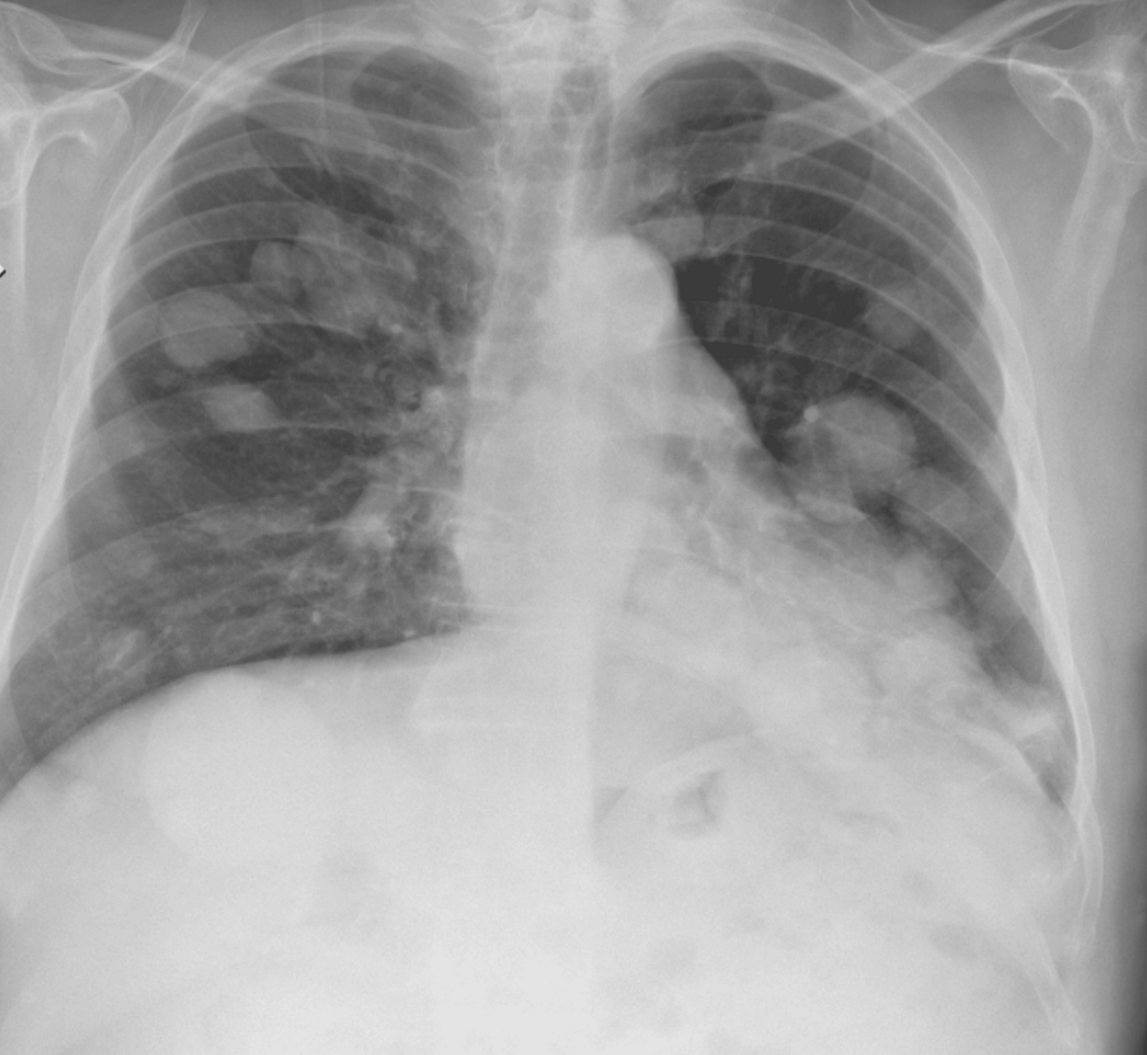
Chest radiograph showing multiple bilateral pulmonary nodules. The radiographic pattern suggests hematogenous spread, raising suspicion for disseminated metastatic disease.
